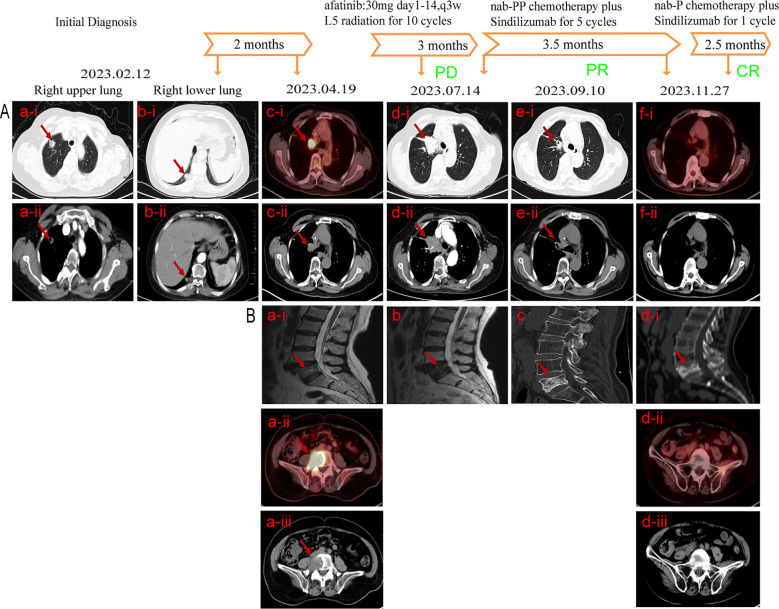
Chest PET/CT/Lumbar MRI/CT images at different time points. (A) Chest CT/PET showing intrapulmonary lesions. [(A), a] Preoperative chest CT showing the lesion in the right upper lung lobe. [(A), b] Preoperative chest CT showing the lesion in the right lower lung lobe. [(A), c] PET-CT on April 19, 2023 showing postoperative recurrence of the right upper lung lesion. [(A), d] Chest CT on July 14, 2023 showing the status of the right upper lung lesion. [(A), e] Chest CT on September 10, 2023 showing the status of the right upper lung lesion. [(A), f] PET-CT on November 27, 2023 showing the status of the right upper lung lesion. (B) CT/PET-CT/MRI showing the metastatic lesions of the L5 vertebral body. [(B), a-i] MRI on April 19, 2023 showing the L5 vertebral metastatic lesion. [(B), a-ii, a-iii] PET-CT on April 19, 2023 showing the L5 vertebral metastatic lesion. [(B), b] MRI on July 14, 2023 showing the L5 vertebral metastatic lesion. [(B), c] CT on September 10, 2023 showing the L5 vertebral metastatic lesion. [(B), d-i] CT on November 27, 2023 showing the L5 vertebral metastatic lesion. [(B), d-ii, d-iii] PET-CT showing the L5 vertebral metastatic lesion (November 27, 2023). CT, Computed tomography; MRI, magnetic resonance imaging; PET, positron emission tomography; PD, Progressive Disease; PR, Partial Response; CR, Complete Response.
